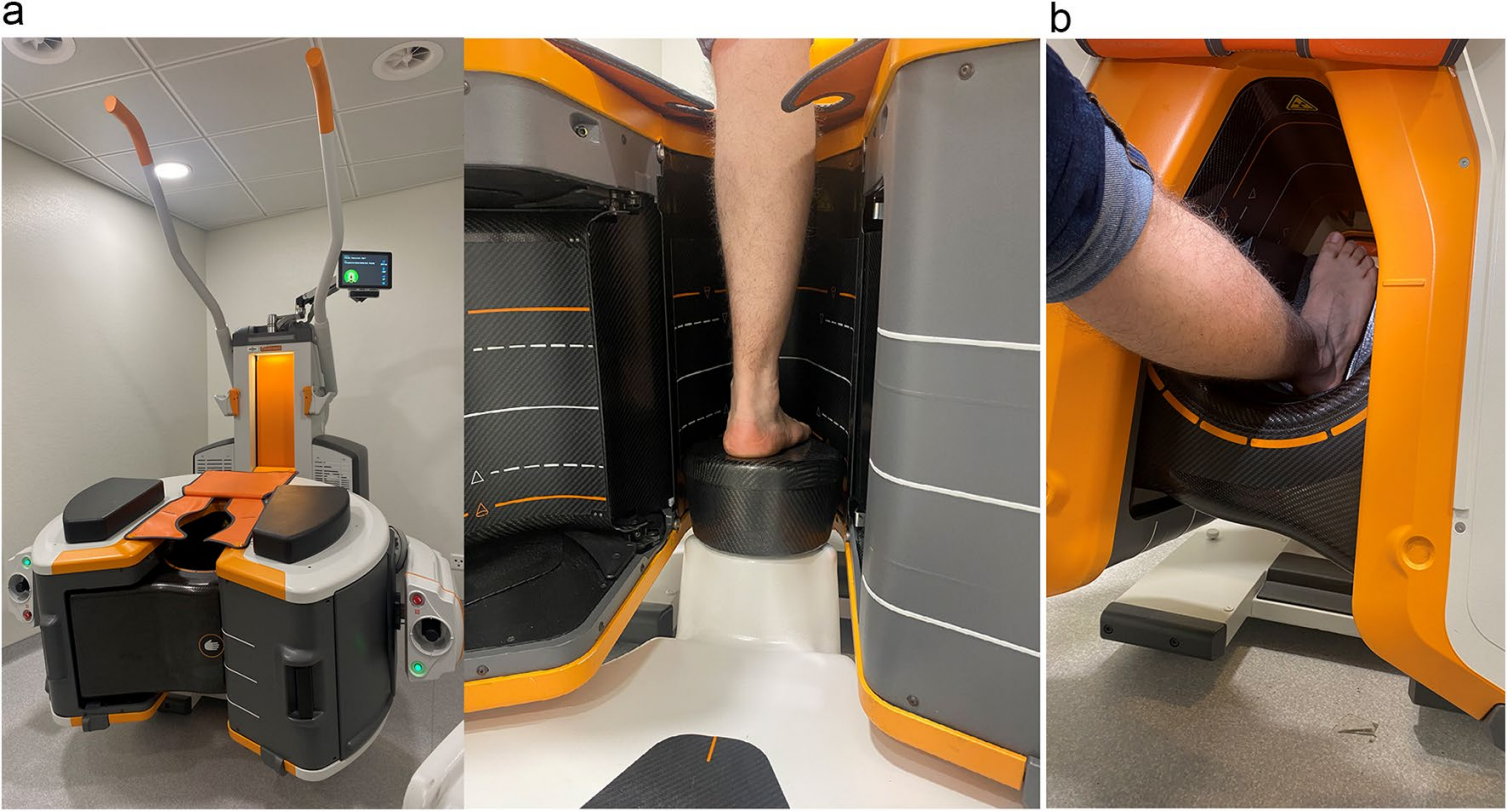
a CBCT in position for acquisition in standing position b or acquisition in a seated position if standing is not possible
